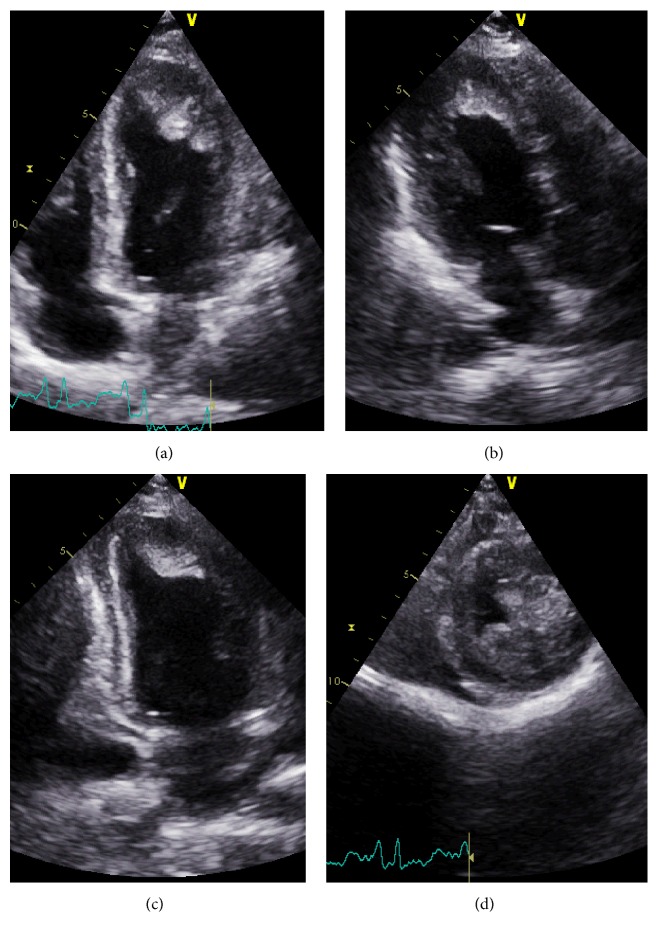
Transthoracic echocardiography showing apical left ventricular thrombus extending to the anterior and anterolateral walls. (a) Apical four-chamber view; (b) apical three-chamber view; (c) apical two-chamber view; (d) short axis view at the apical level.
